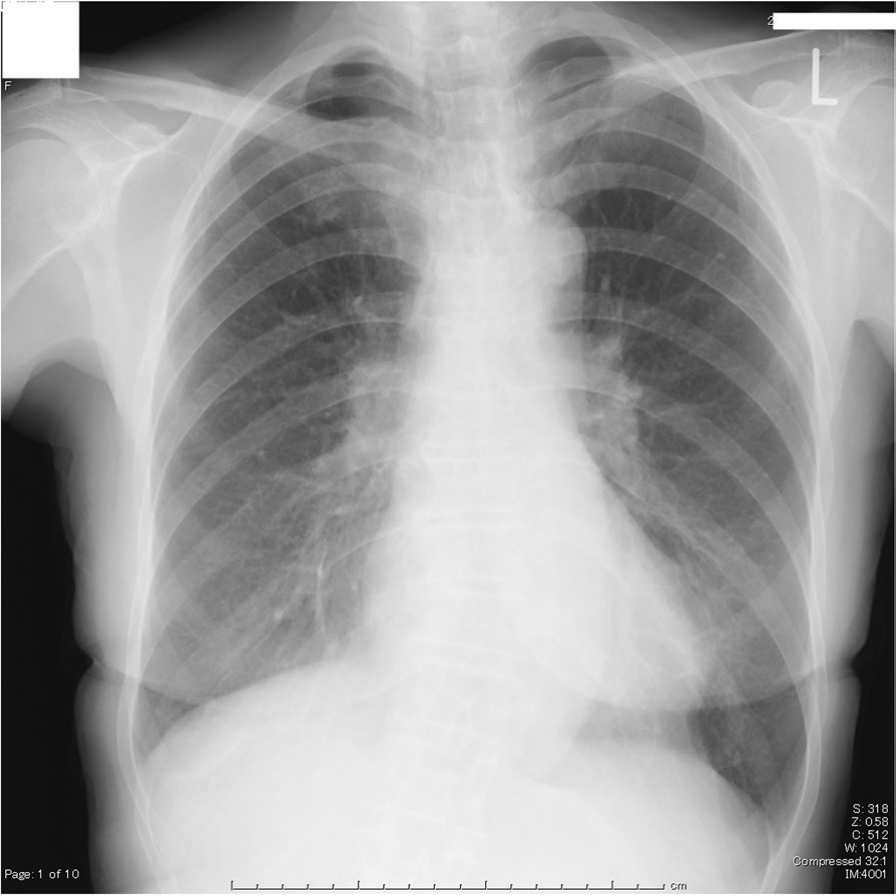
Preoperative chest X-ray did not show any abnormalities
